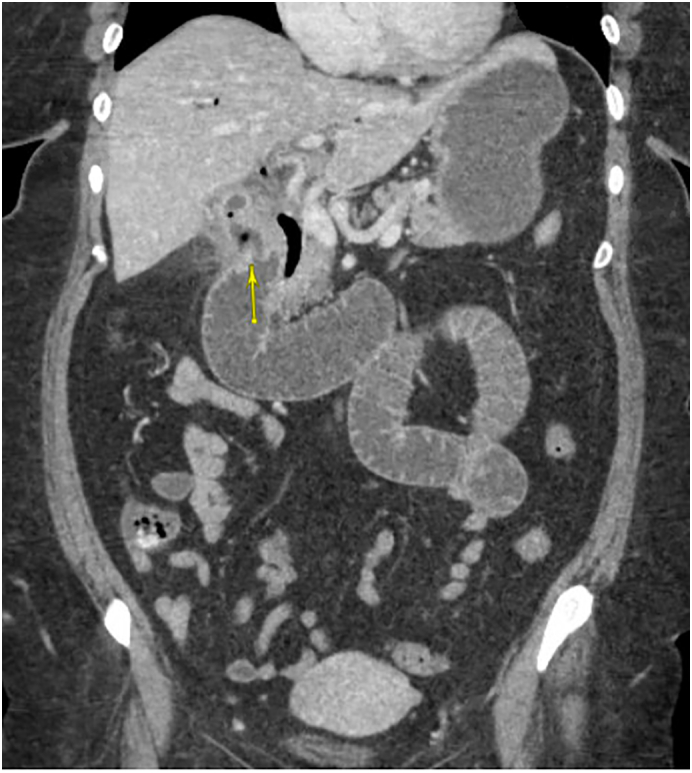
Computed tomography of the abdomen showing pneumobilia and a fistulous track between the gallbladder and duodenum.
